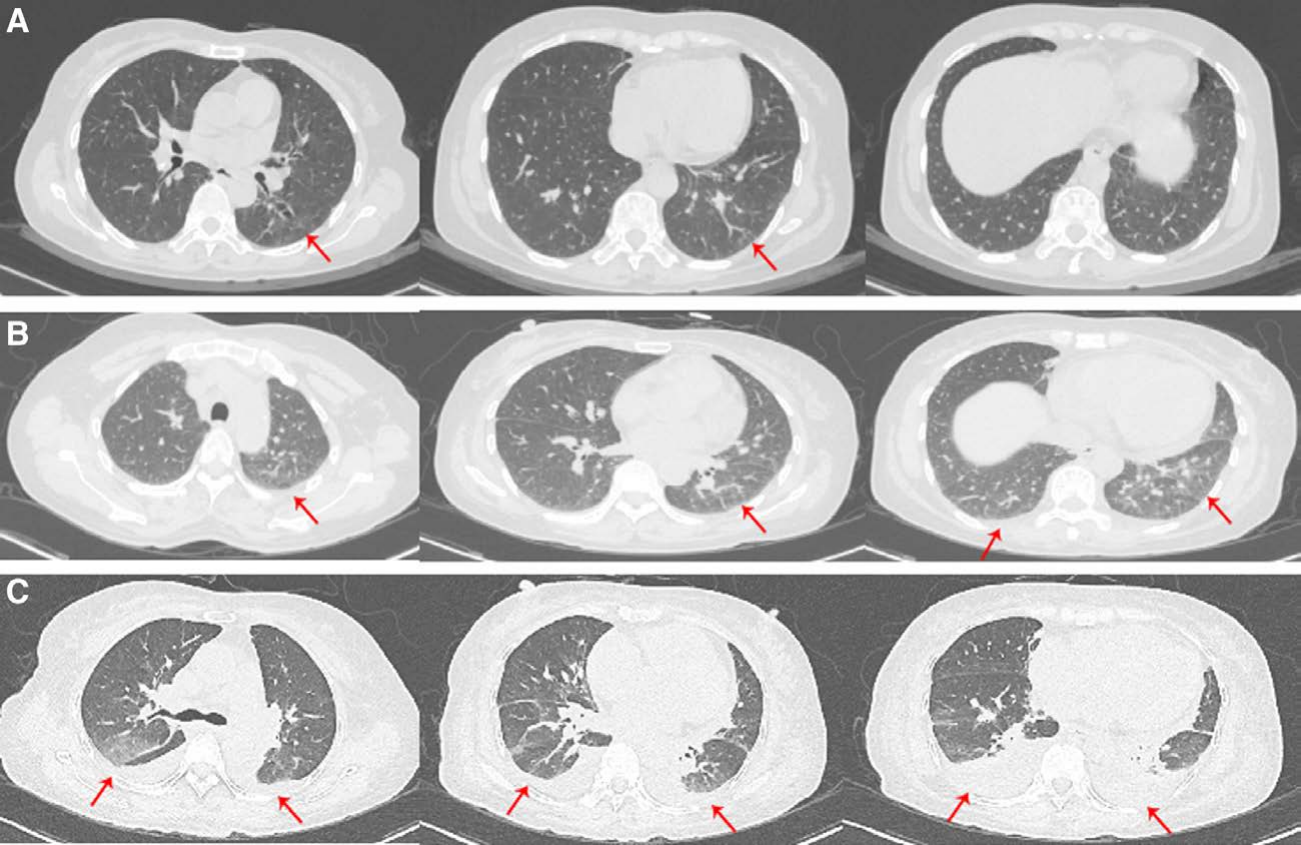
Chest CT images of the patient in the early stages of the disease. (A) CT image of the patient on October 2, arrows showed a few exudative lesions in both lungs. (B) CT image of the patient on October 21, arrows showed slightly increased exudative lesions in both lungs. (C) CT image of patient November 3, arrows showed significantly increased ground-glass lesions in both lungs and new pleural effusion.
